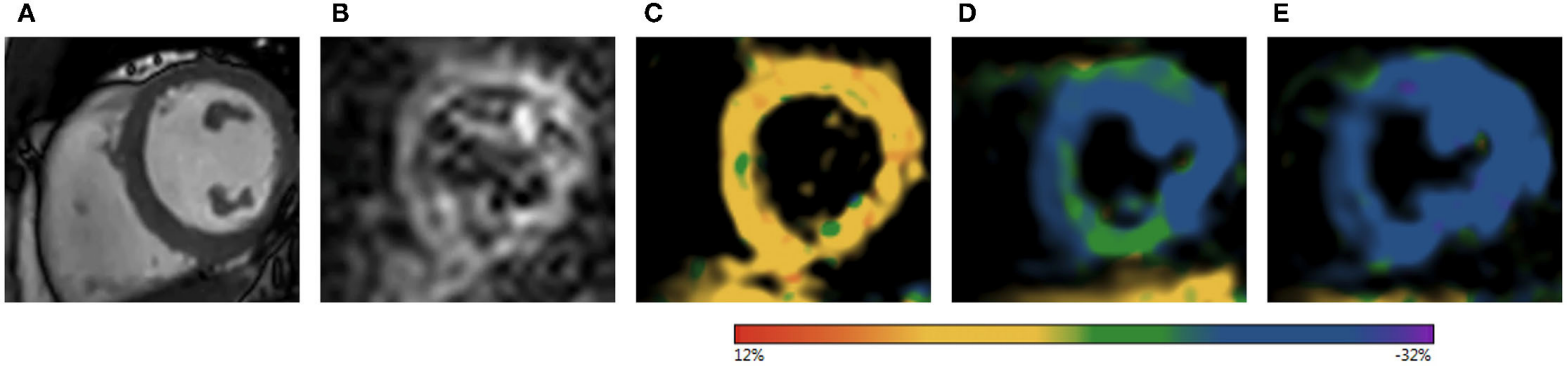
Example of the image acquisition and GLS response of a healthy volunteer as visualized in the MyoStrain® software. (A) Cine sequence of a midventricular short axis slice. (B) fSENC-images of midventricular short axis slice at end-diastole. (C–E) Corresponding color-coded images after post-processing at end-diastole (C), end-systole at rest (D), and after 2 min of DHE (E). As demonstrated in (D,E), peak systolic longitudinal strain increased after DHE (color scale represents regional longitudinal strain). GLS, global longitudinal strain; fSENC, fast strain-encoded magnetic resonance imaging; DHE, dynamic handgrip exercise.
